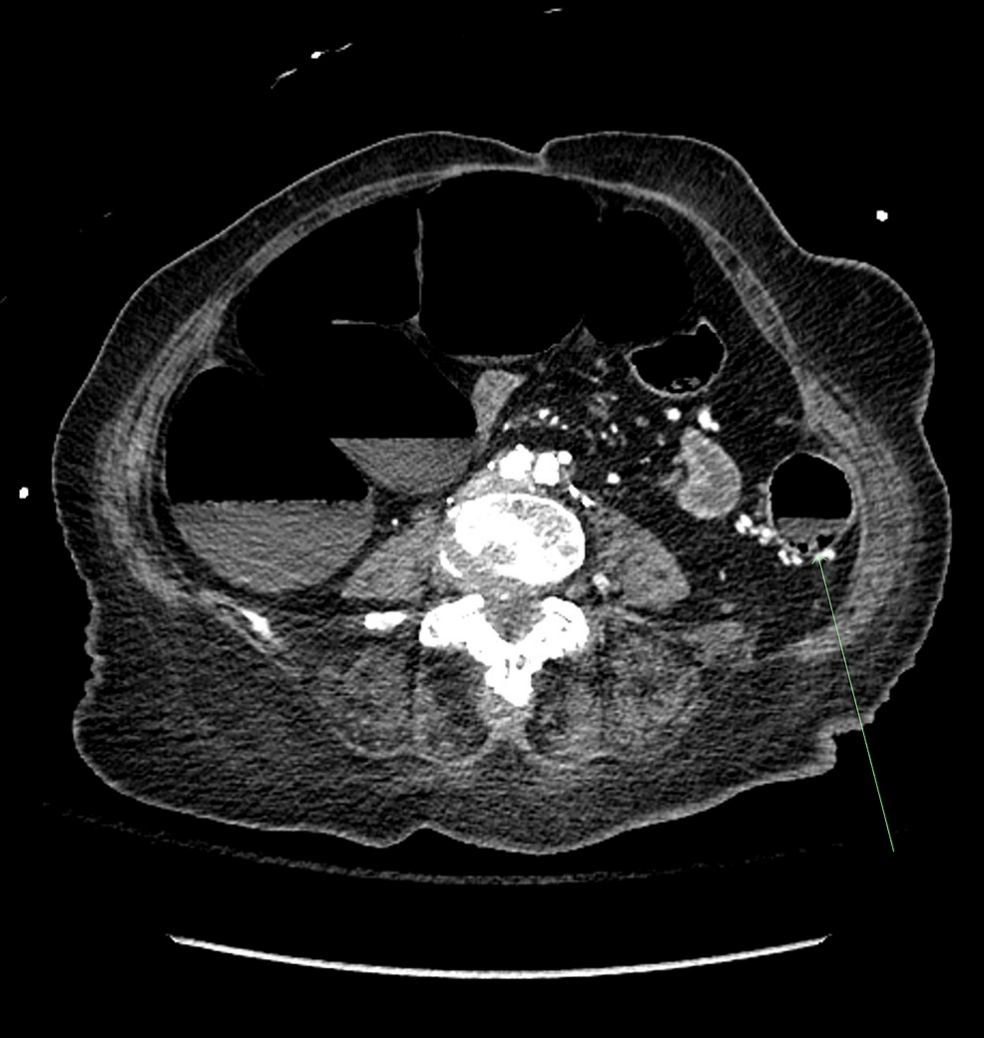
Axial view CT with dilated loops of bowel with arrow noting the area of pneumatosis.
